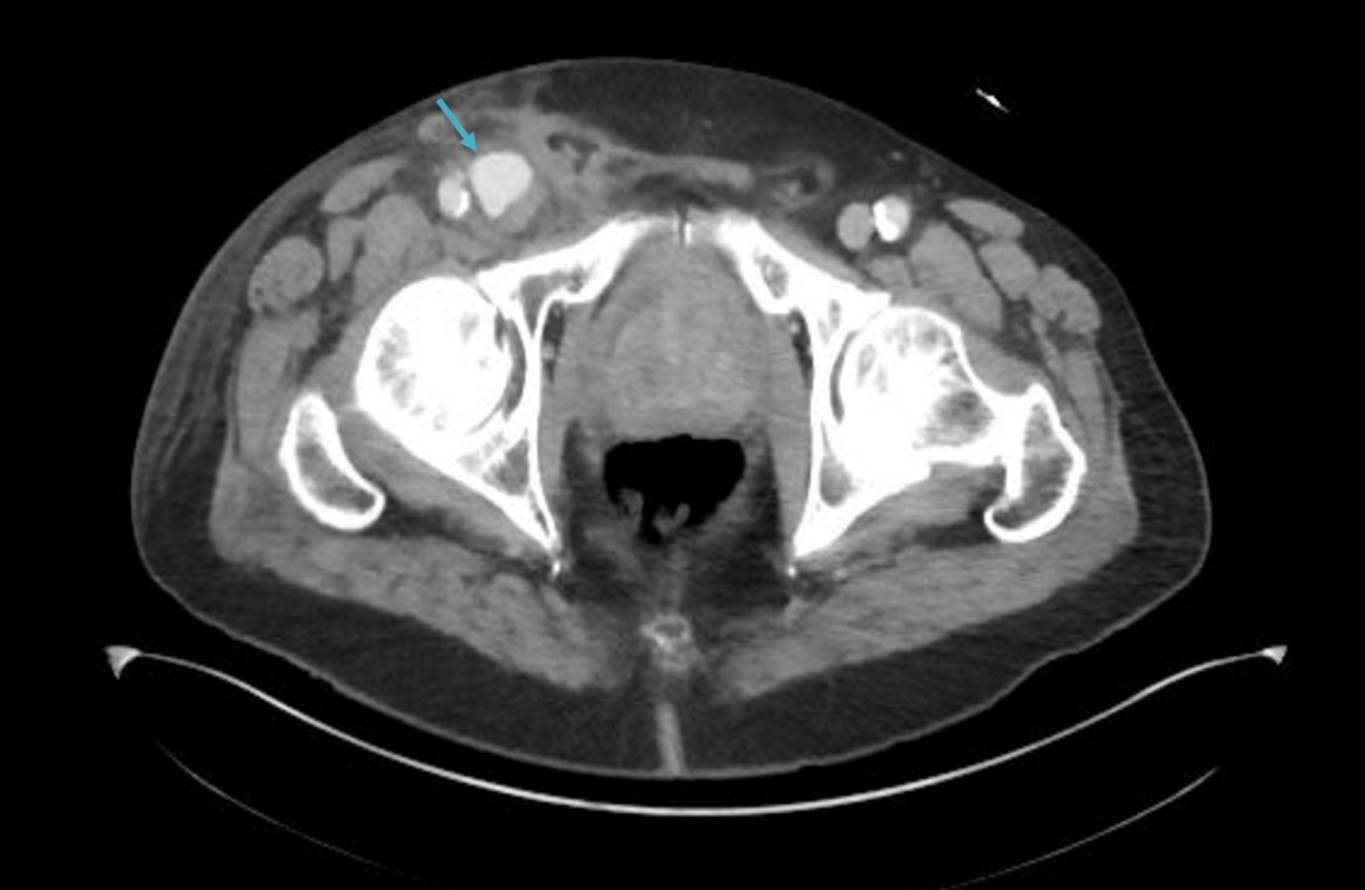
Computed tomography scan of the pelvis showing pseudoaneurysm of the right common femoral artery (arrow)
